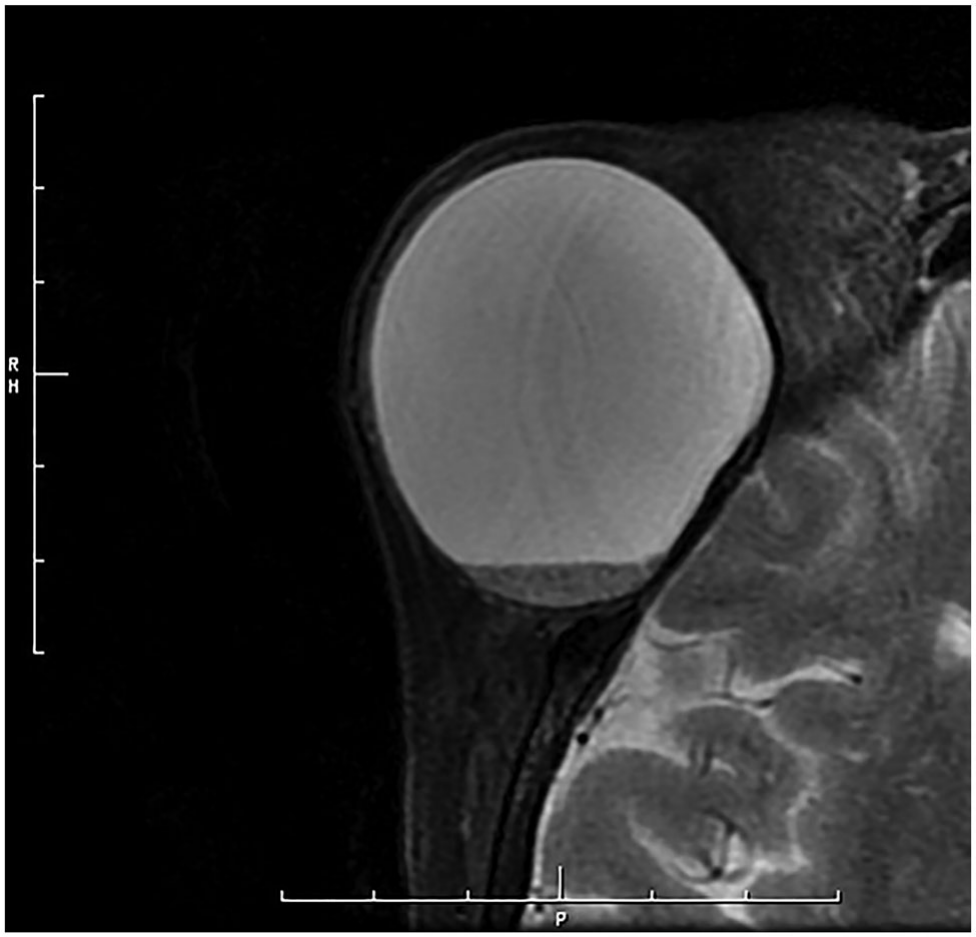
T2-weighted MRI demonstrates a round, high-intensity mass.
